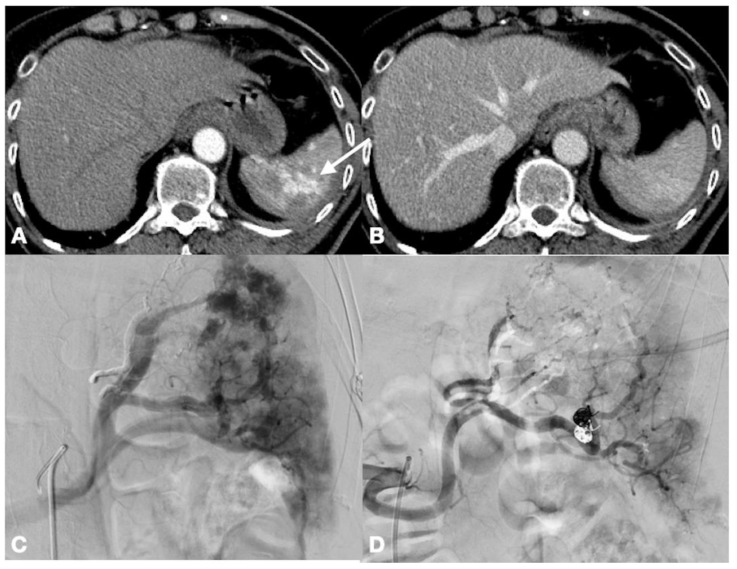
Enhanced-CT of a 56-year old male who sustained major trauma. Arterial (A) and portal venous phase (B). There is a small volume hemoperitoneum and multiple contained vascular injuries (A, arrow) that can be seen only in the arterial phase. The patient underwent angiography which confirmed CT findings (C), followed by successful embolization (D).
